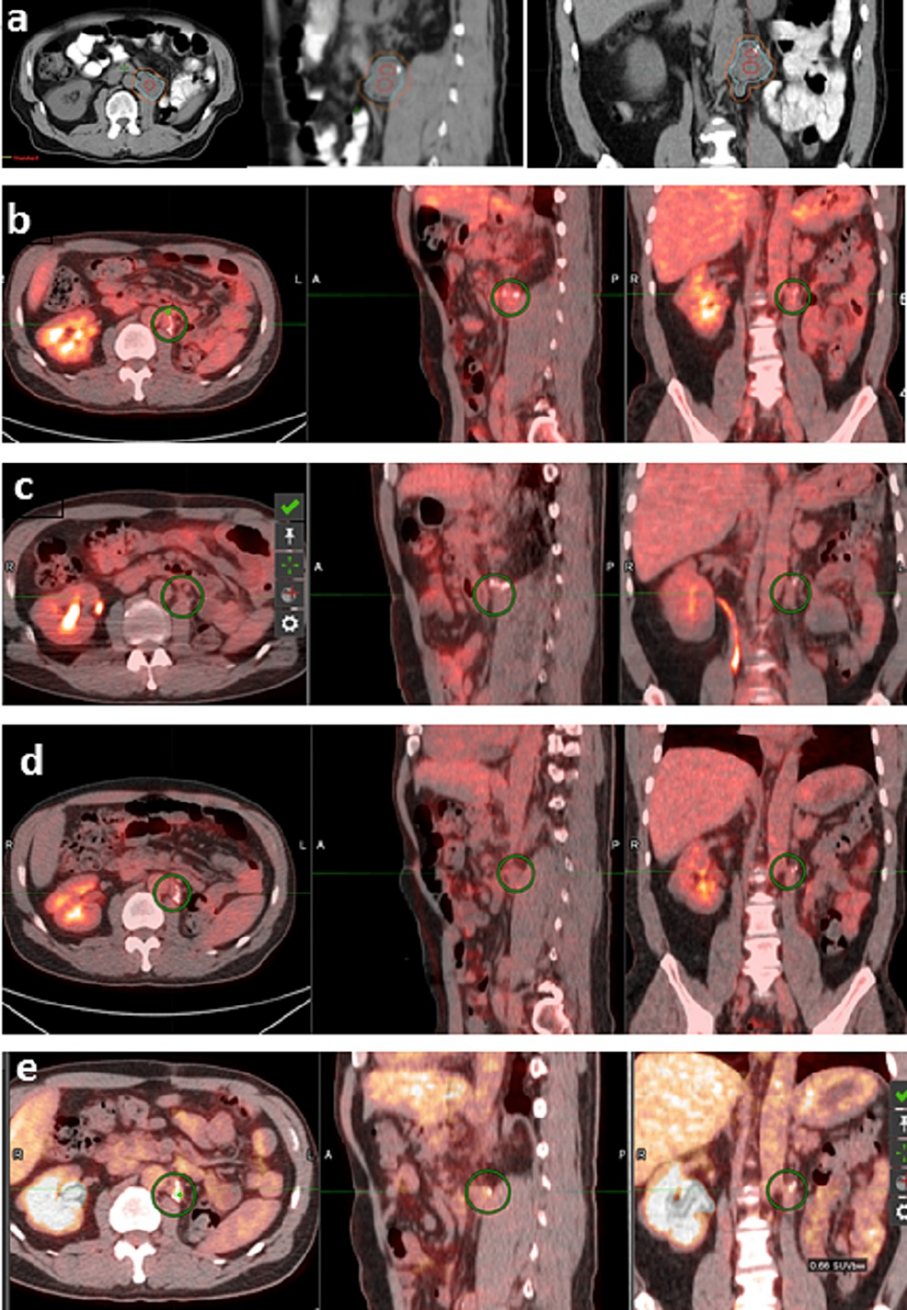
Sequential decrease in tumor size from PET-CT image fusions (axial, sagittal, and coronal) around the irradiated bed during the follow-ups. a: June 2022 treatment planning CT, b: August 2022 post-treatment, c: November 2022, d: February 2023, e: June 2023 PET-CT: positron emission tomography-computed tomography
